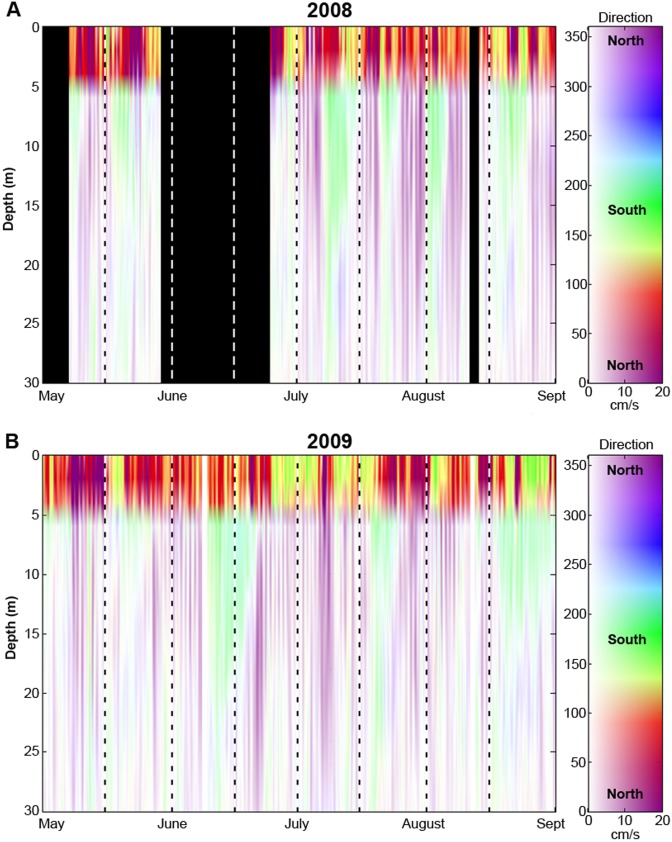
Acoustic Doppler Current Profiler (ADCP) data from 2008 (Fig. 5A) and 2009 (Fig. 5B). Purple colors indicate northerly flowing currents, green colors indicate southerly flowing currents, and red colors indicate onshore flow. Intensity of color represents magnitude of flow. Black regions represent periods of time when ADCPs were not collecting data.
